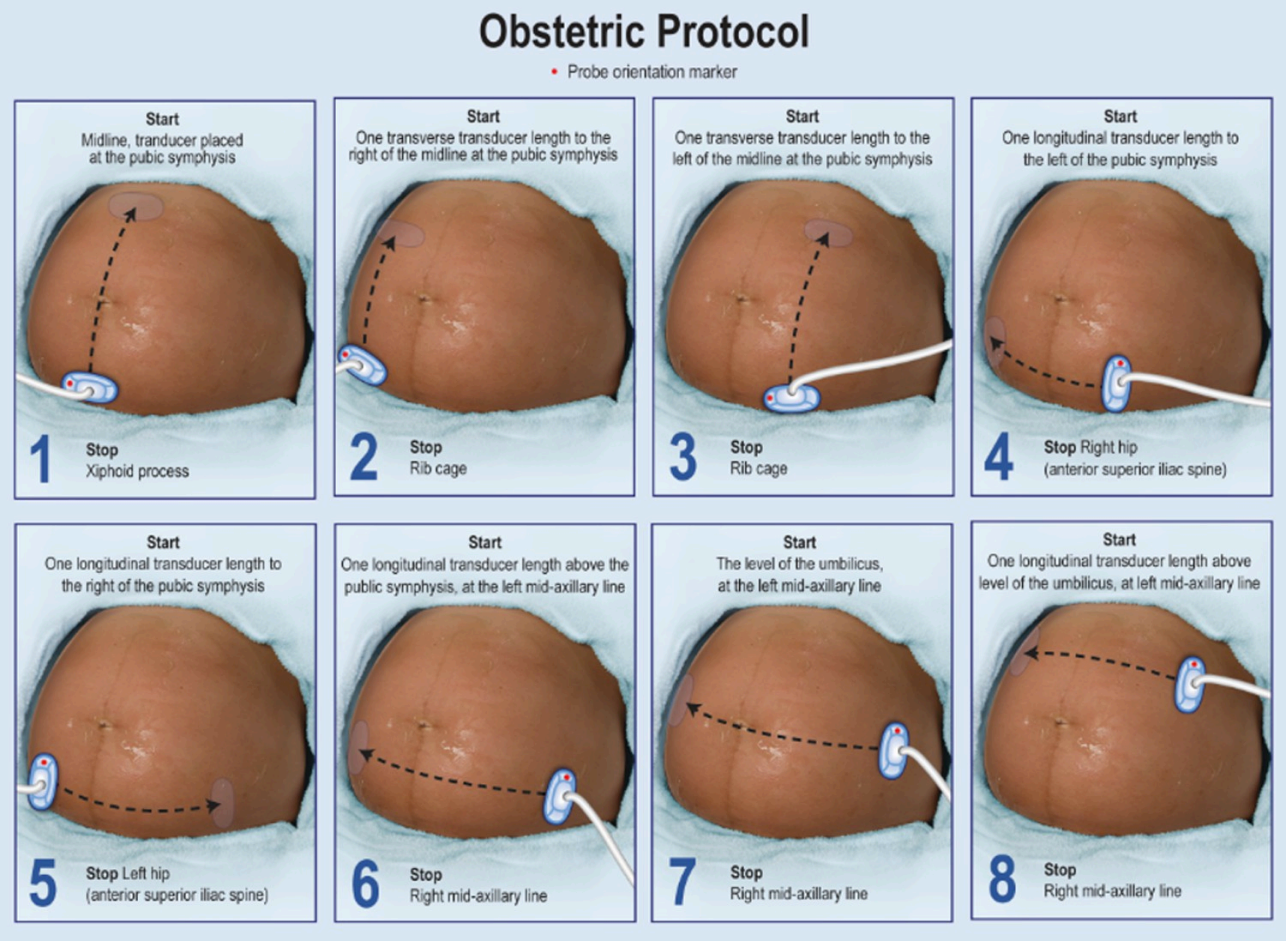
Obstetric ultrasound volume sweep imaging protocol. Poster depicting each step in the VSI protocol.
